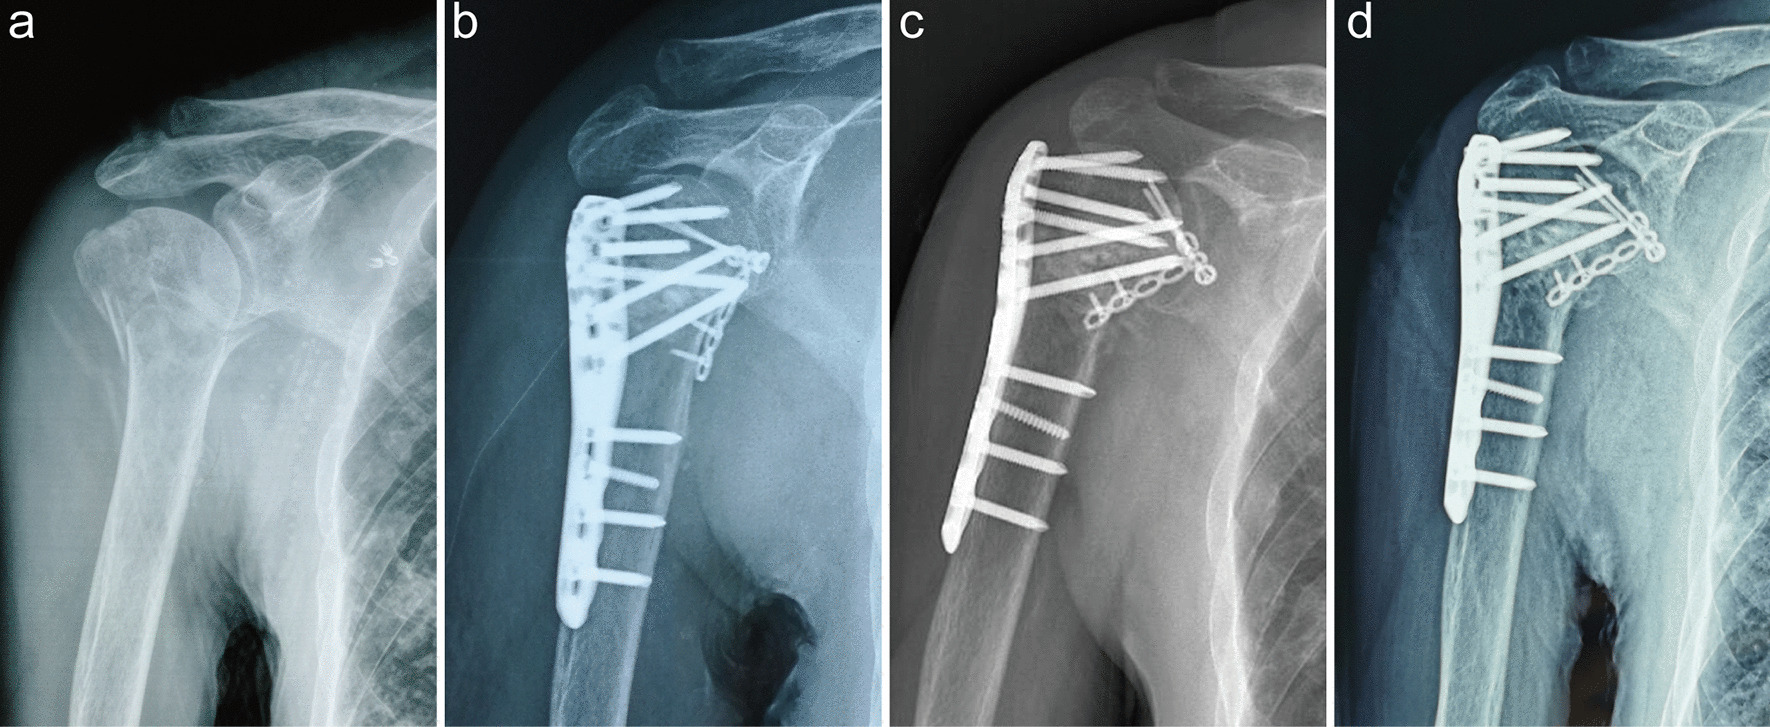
A 69-year-old patient with avascular necrosis of the humeral head. The AP (a) radiograph showing a Neer 3-part proximal humeral fracture. This patient was treated with PHILOS combined with a small anteromedial locking plate (b). The X-ray images obtained at 11 weeks after the surgery showing bony union of the fracture (c). Avascular necrosis of the humeral head and screw penetration were observed 14 months after the surgery (d)
